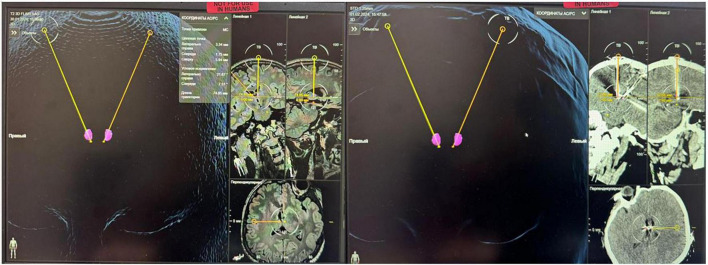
The electrode placement site was confirmed by postoperative CT scan of the alignments on Brainlab with preoperative planning.
